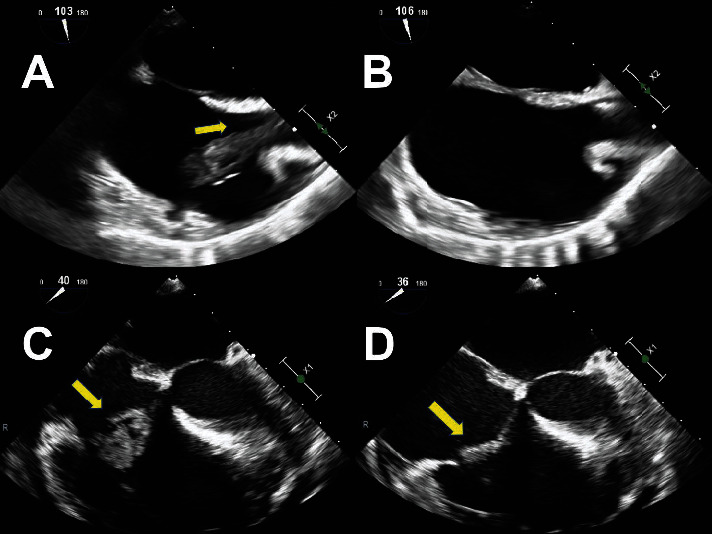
Pre- and postprocedural transesophageal echocardiographic imaging of the removal of large thrombi via AngioVac. (a, c) Large vegetation across the mitral valve. (b, d) Near removal of multisegmented vegetation. There is good intraoperative success.
